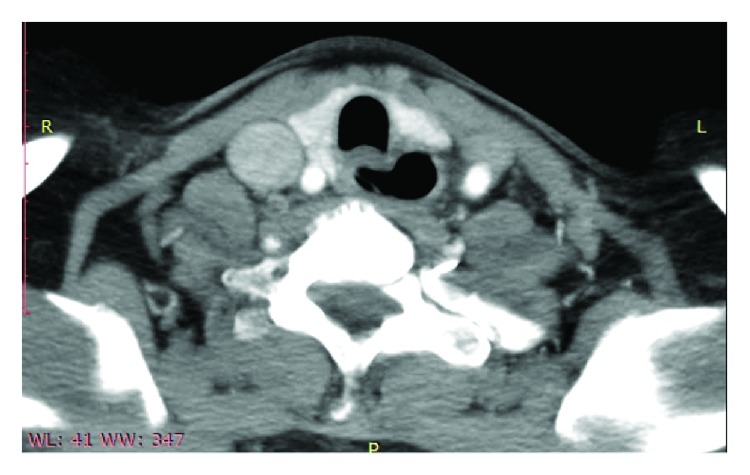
Axial CT scan image of the neck with contrast confirming the diverticulum arising from the midline that is directed towards the left side.
